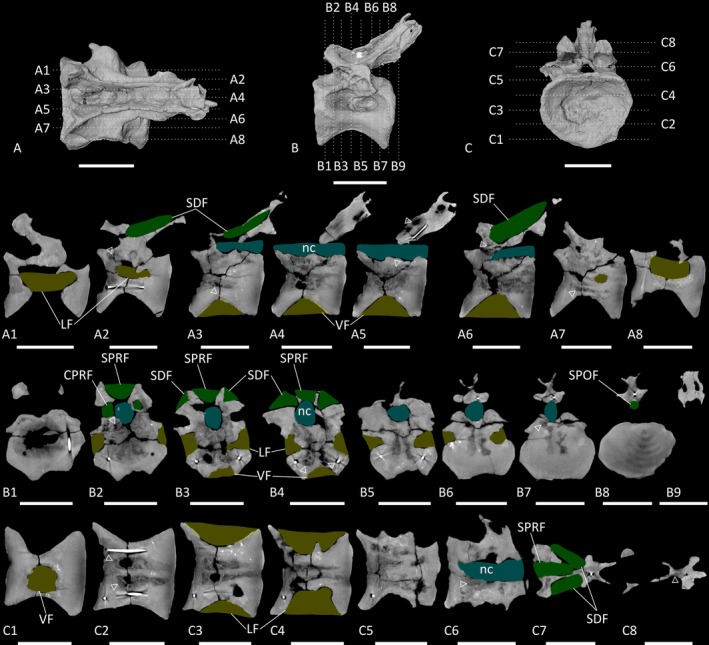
CT scan of *Tornieria africana* middle caudal vertebra MB.R.2956.5. (A–C) 3D rendering (A) dorsal view, (B) left lateral view, (C) anterior view; (A1–A8) CT scan slices in parasagittal cross‐section, (B1–B9) CT scan slices in transverse cross‐section, (C1–C8) CT scan slices in horizontal cross‐section. CPRF, centroprezygapophyseal fossa; LF, lateral fossa; nc, neural canal; SDF, spinodiapophyseal fossa; SPOF, spinopostzygapophyseal fossa; SPRF, spinoprezygapophyseal fossa; VF, ventral fossa. Key: Blue highlight, neural canal; green highlight, neural spine fossa; yellow highlight, centrum fossa; white arrow, pneumatic bone texture. Scale bars = 100 mm.
